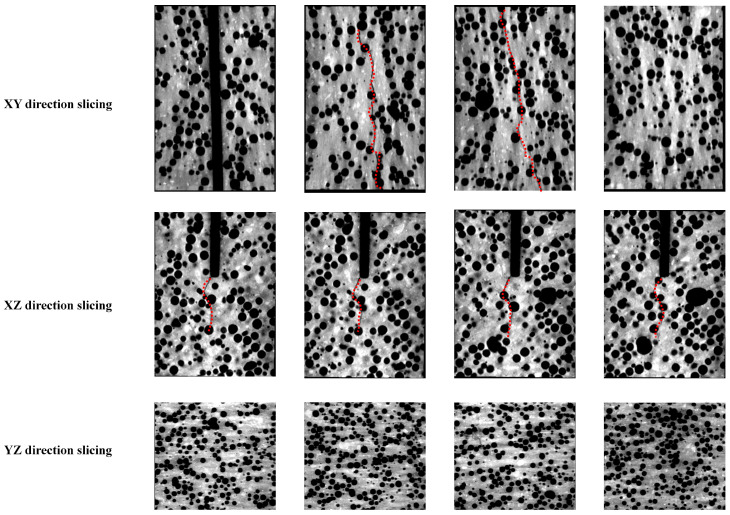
Sections in all directions under CT scanning. Where the red dotted line indicates the path of development of the cracks.
